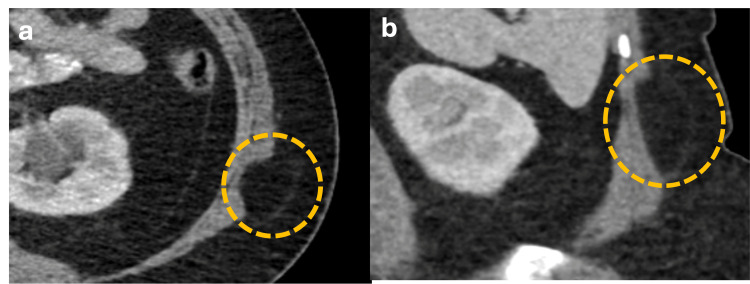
Axial and coronal abdominal CT scan showing an abdominal wall lipoma (a, b) Axial and coronal CT scan showing a hypodense well defined fatty mass within the abdominal wall (yellow dotted circle), consistent with a lipoma. The figure was created by the authors.
